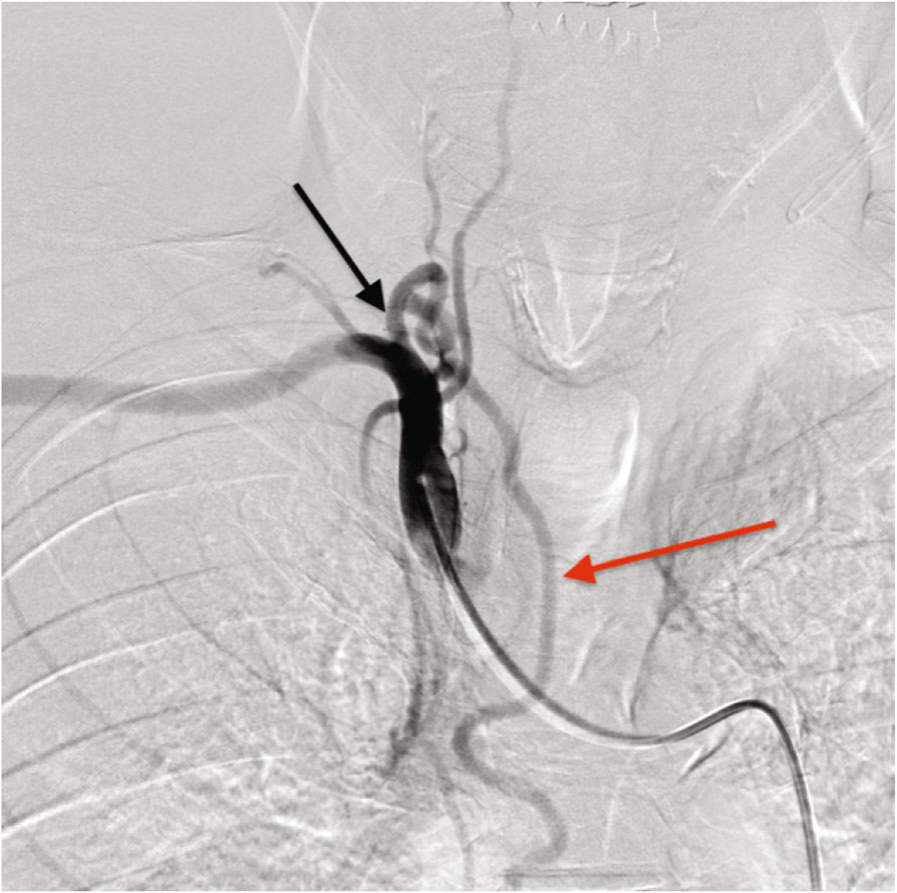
Angiogram of the brachiocephalic artery shows the hypertrophic ectopic bronchial artery (red arrow) arising from the right thyrocervical trunk (black arrow), findings that corresponded with the CTA image
